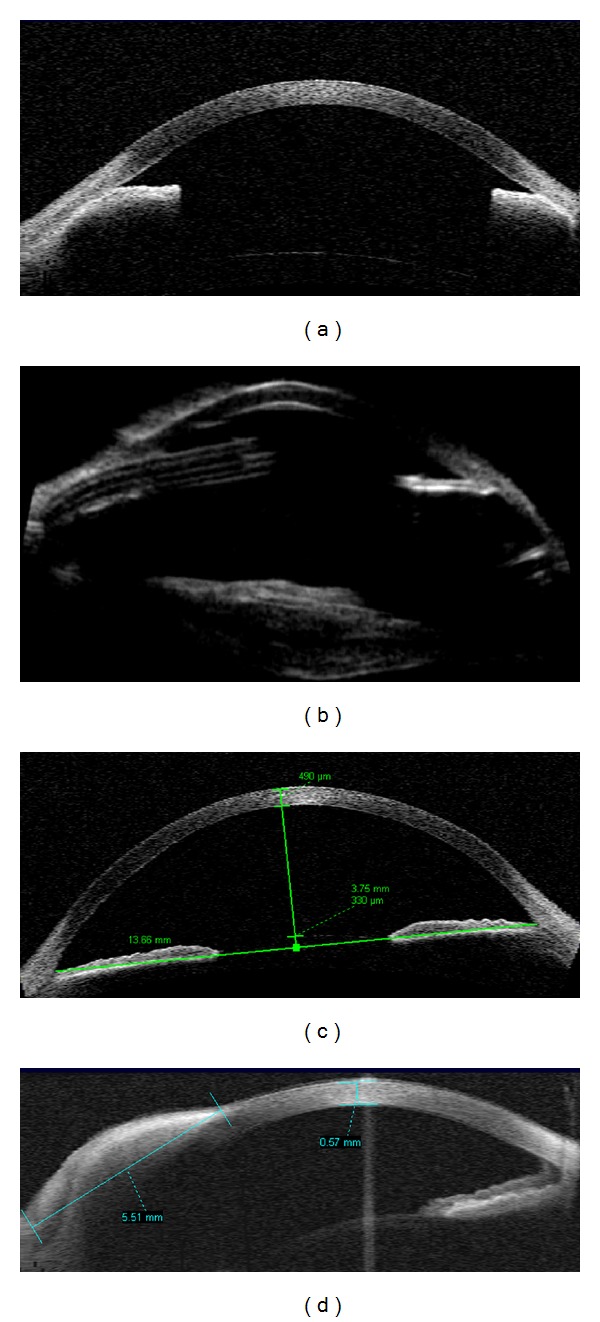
(a) Anterior segment OCT in pediatric patients. Neovascular glaucoma and secondary angle closure (anterior synechiae). (b) High frequency ultrasound (UBM) of the same eye after implantation of glaucoma drainage tube, showing the tube in the anterior chamber, an irregular and hyperrefletive lens and angle closure at the opposite position (c) AS-OCT in a congenital glaucoma with anterior chamber depth = 3.75 mm. (d) Corneo-scleral dermoid cyst with posterior attenuation, radial extension = 5.51 mm.
